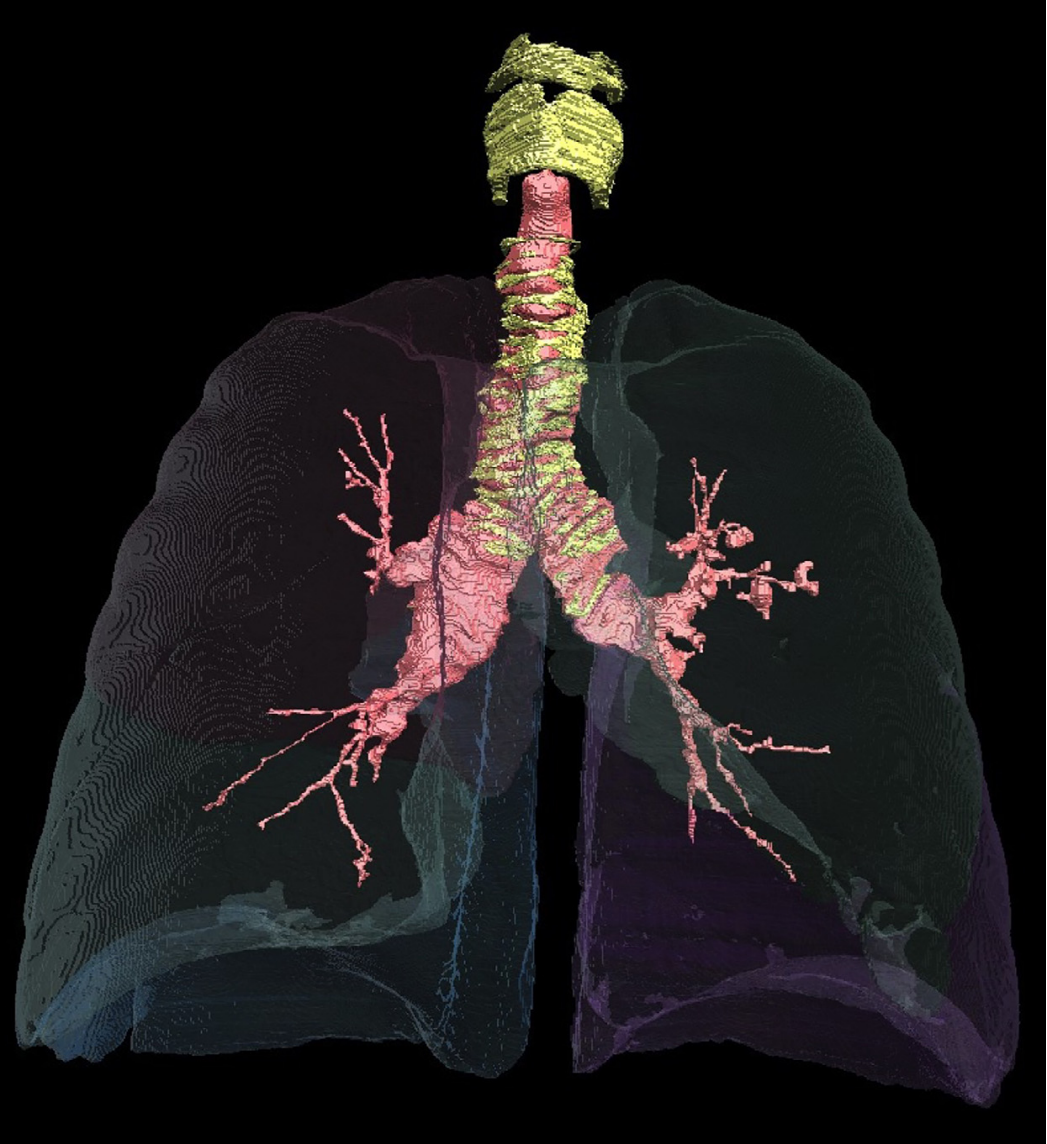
CT‐based 3D reconstruction of airway tree from an adult with Mounier–Kuhn syndrome. Airways are outlined in pink and cartilage in yellow.
